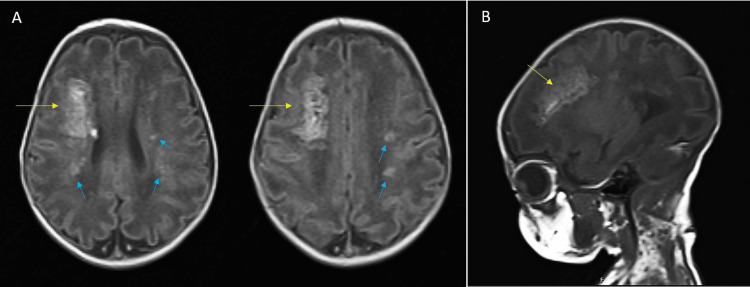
Brain MRI of twin A performed on the sixth day of life The axial T2-weighted view (A) and parasagittal T2-weighted view (B). The scans reveal thrombosis of the deep medullary veins in the right frontoparietal lobe (yellow arrows), along with additional small regions of ischemia (blue arrows), involving both internal capsules
